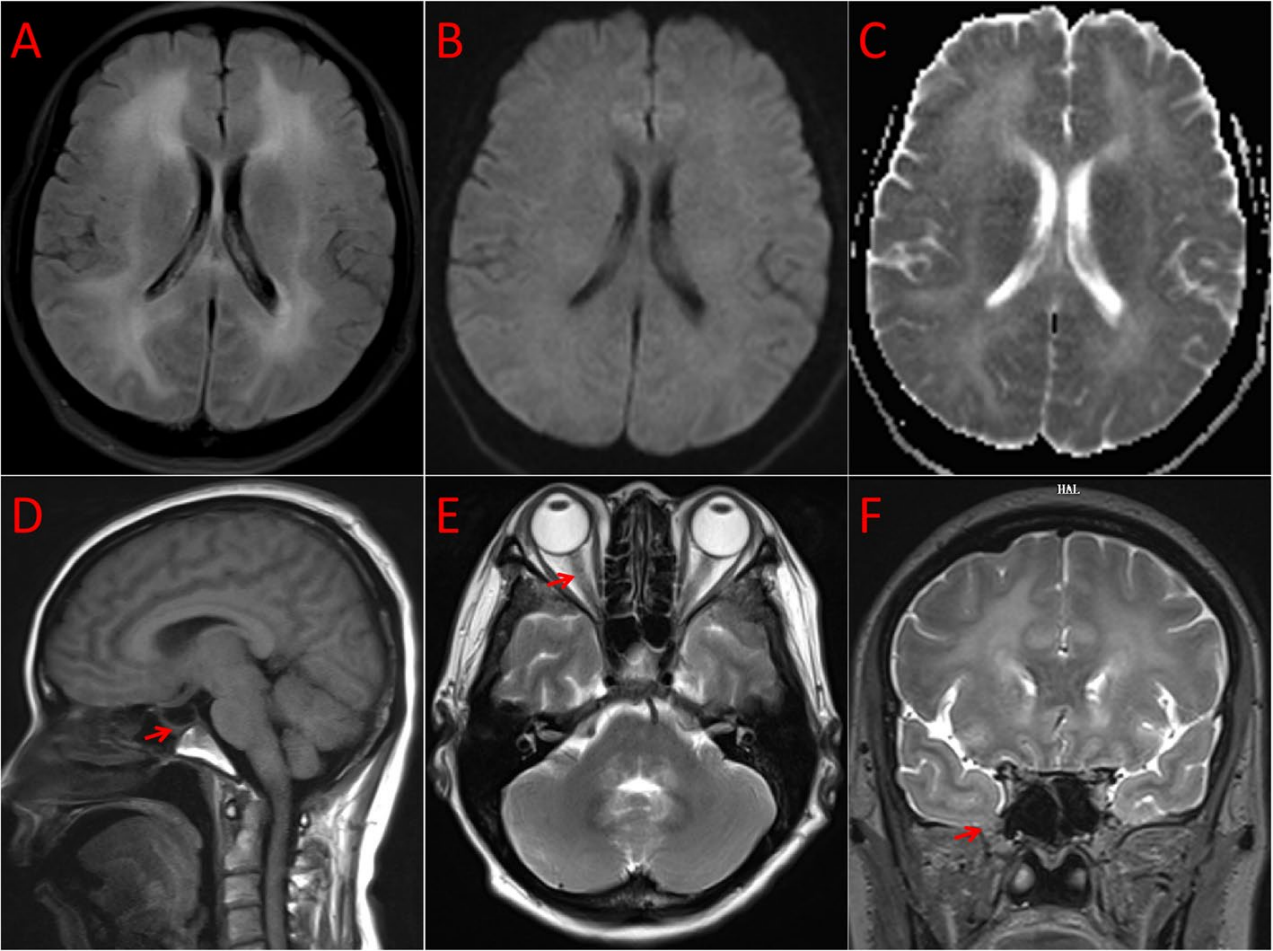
MRI results of the patient. The MRI showed a high FLAIR signal, a
